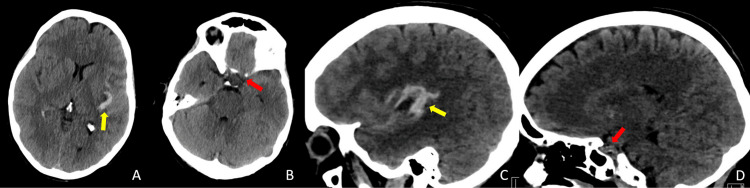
Emergent Head CT (October 2020) Emergent Head CT (October 2020) indicative of an acute subarachnoid hemorrhage involving the left Sylvian fissure extending to the left frontal and temporal sulci (A axial and C sagittal images). No hemorrhagic extension within the basal cisterns was noted (B axial and D sagittal images)—yellow arrow indicating the SAH distribution. The red arrow was used as a reference to define where they would have been located the SAH in case of the left ICA aneurysm rupture.
